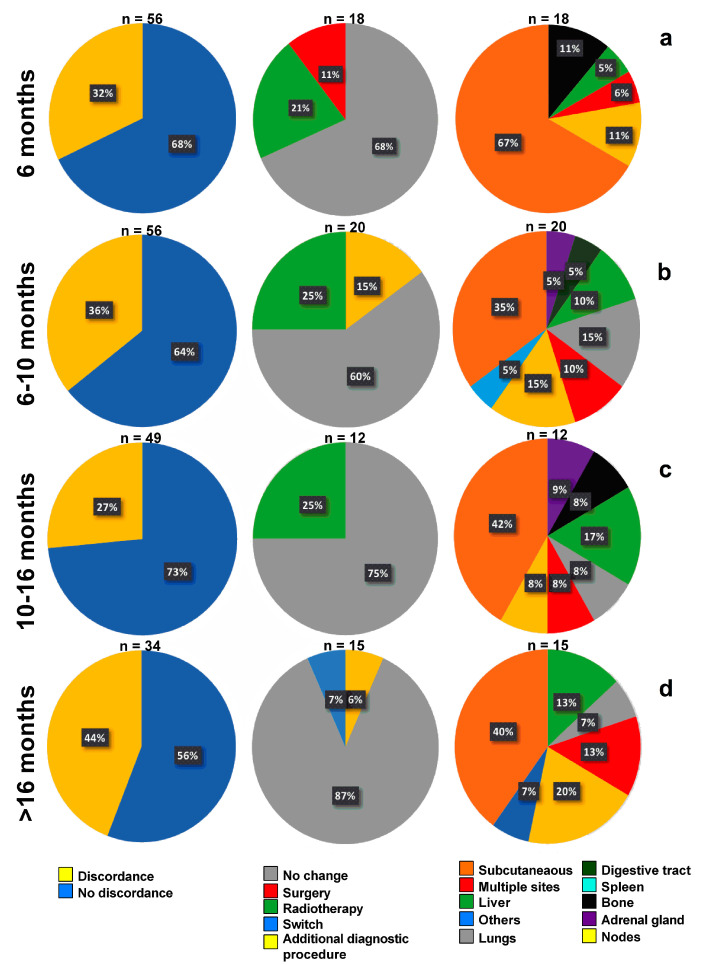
From left to right: repartition of discordance in imaging response between ^18^ F-FDG PET/CT and ceCT, induced changes in patient’s management, and anatomical site responsible for the observed discordances. Data are categorized based on the time elapsed since introduction of treatment (defined as quartiles) (a) early evaluation, (b,c) interim evaluation, and (d) late evaluation.
